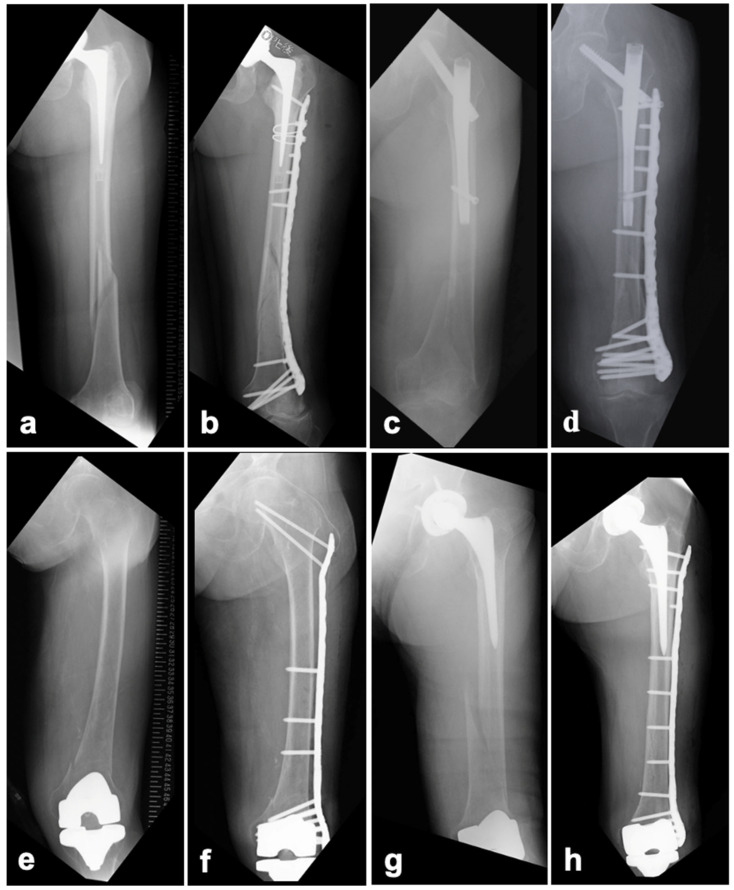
WSP in cases involving peri-BHA/THA, peri-SFN, peri-TKA, and interprosthetic femoral fractures. Preoperative (a,c,e,g) and postoperative (b,d,f,h) radiographic images.
